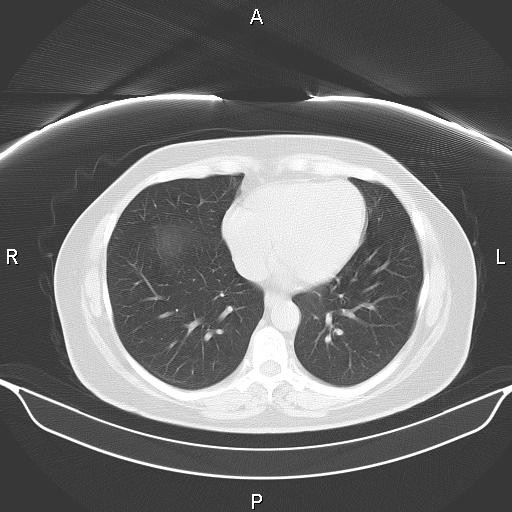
Computed tomography of the thorax. Thorax CT was normal on admission to the ICU, indicating the absence of overt pulmonary involvement.
